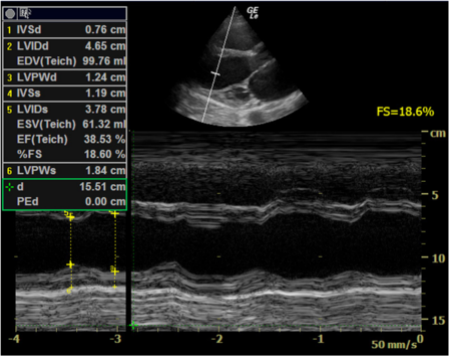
Poor LV systolic function. This parasternal long axis view shows a dilated LV with poor fractional shortening (FS = 18.6 %) in a patient with dilated cardiomyopathy and hypotension (*FS* = fractional shortening)
